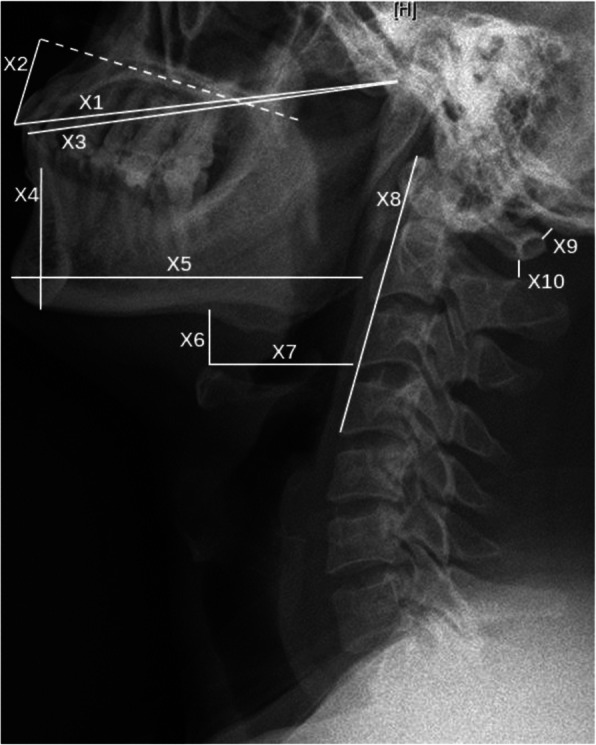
Distance indicators on the lateral cervical X-ray in the neutral position. X1: distance between temporomandibular joint and the tip of upper incisor; X2: perpendicular distance from hard palate to the tip of upper incisor; X3: distance between temporomandibular joint and the tip of lower incisor; X4: anterior depth of mandible; X5: length of mandibular body; X6: vertical distance from the highest point of hyoid bone to mandibular body; X7: horizontal distance from the highest point of hyoid bone to the border of the nearest cervical vertebra; X8: distance from the anterior-inferior border of the fourth cervical vertebra to the anterior-superior border of the first vertebra; X9: atlanto-occipital gap; X10: distance between the spinous processes of the first and second cervical vertebra
